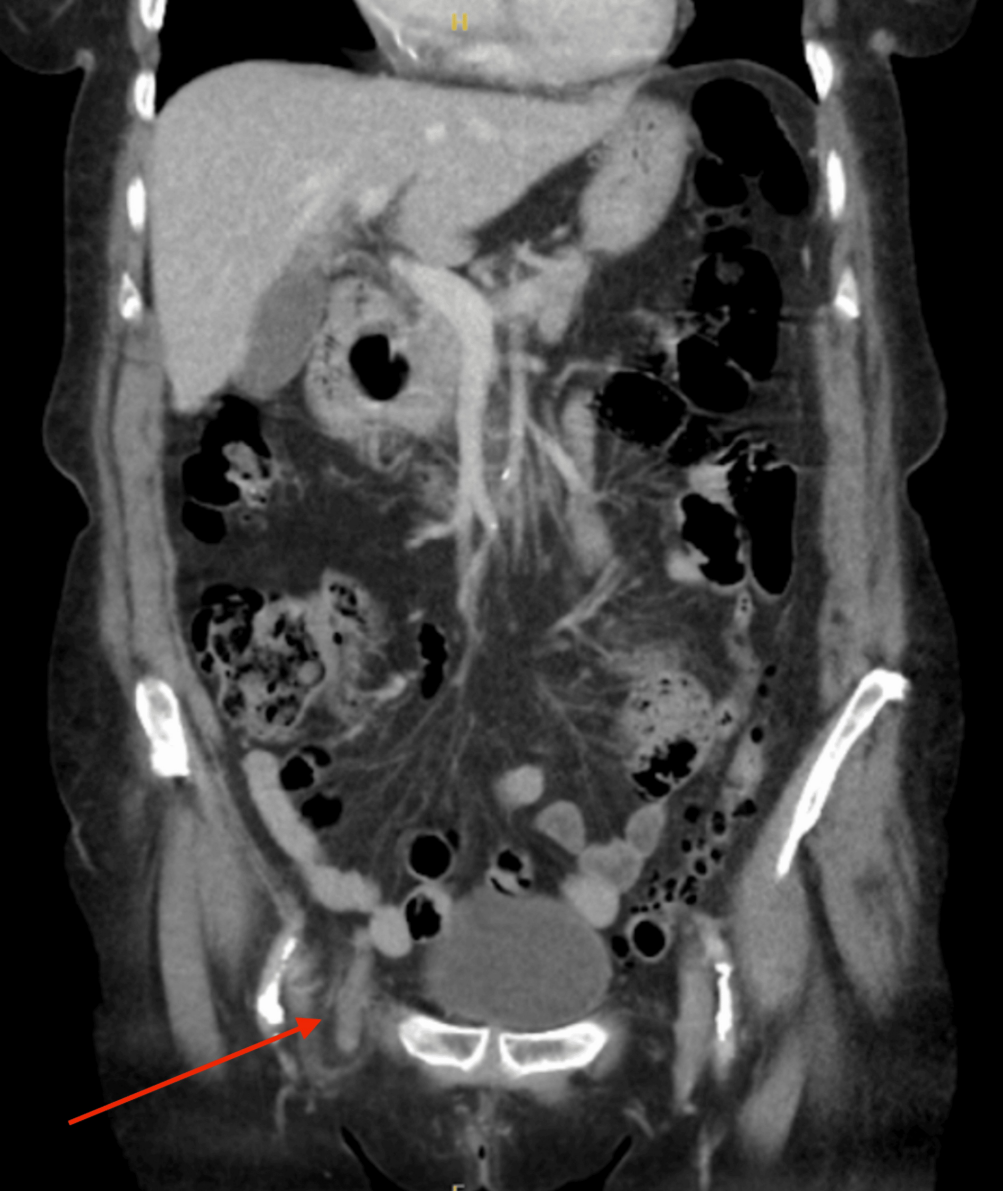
A coronal section of a CT scan showing an inflamed appendix within a right-sided femoral hernia, indicated by an arrow
